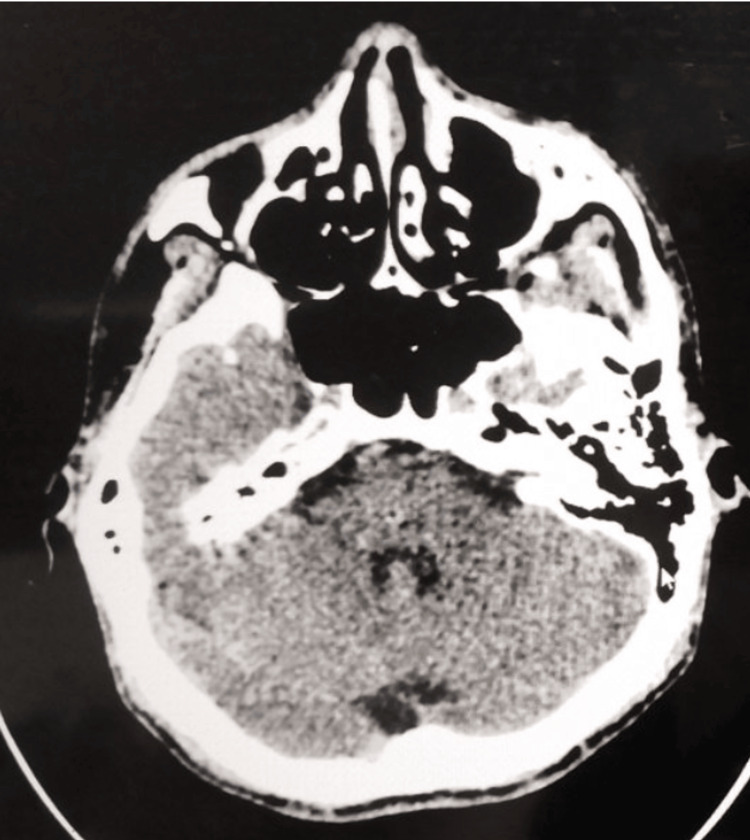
Plain cranial CT scan axial view on day 28 post-ictus, demonstrating resolution of the pontine hemorrhage.
